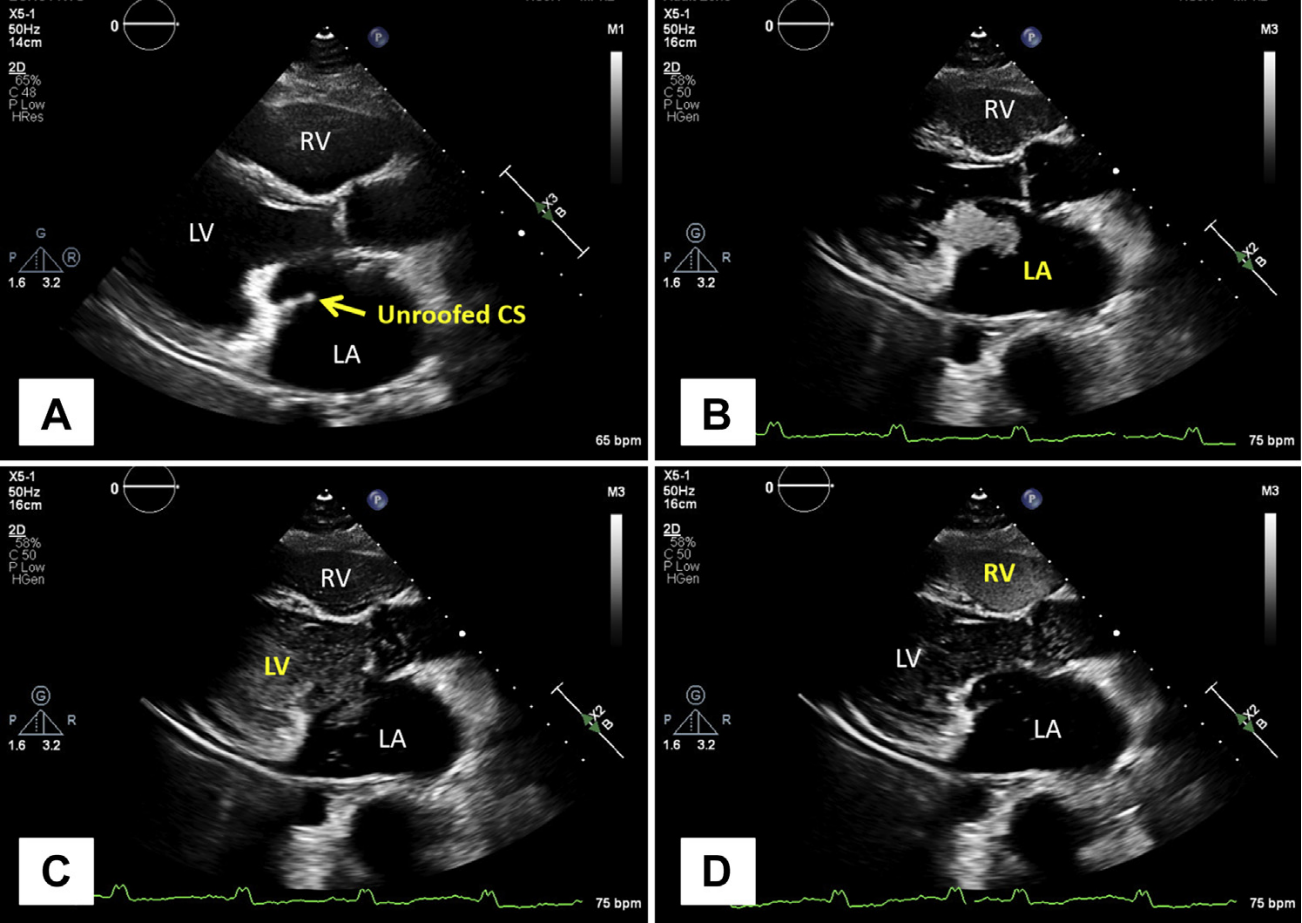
Case 3: persistent L-SVC, unroofed CS. Transthoracic echocardiogram in parasternal long-axis view with intravenous agitated saline injection in the left arm. The continuity between the CS and LA (A) confirms the presence of an unroofed CS. After injection of agitated saline into the patient's left arm, the CS opacifies first (B), followed by the left heart (C) and the right heart (D). The opacification of the CS first is diagnostic of an L-SVC, while the opacification of the left heart before the right heart confirms the unroofed CS. The co-occurrence of these 2 findings is also known as Raghib syndrome. Video 4 corresponds to this figure. *CS*, Coronary sinus; *RV*, right ventricle.
